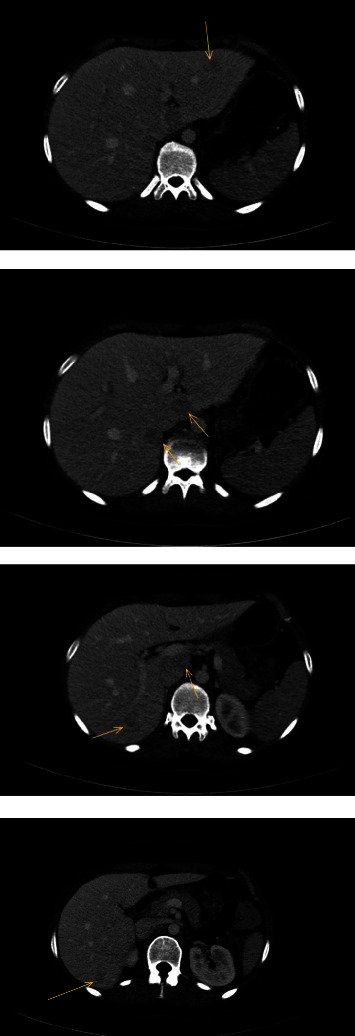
Liver CT demonstrated the onset of seven liver lesions suspect for metastatic disease.
